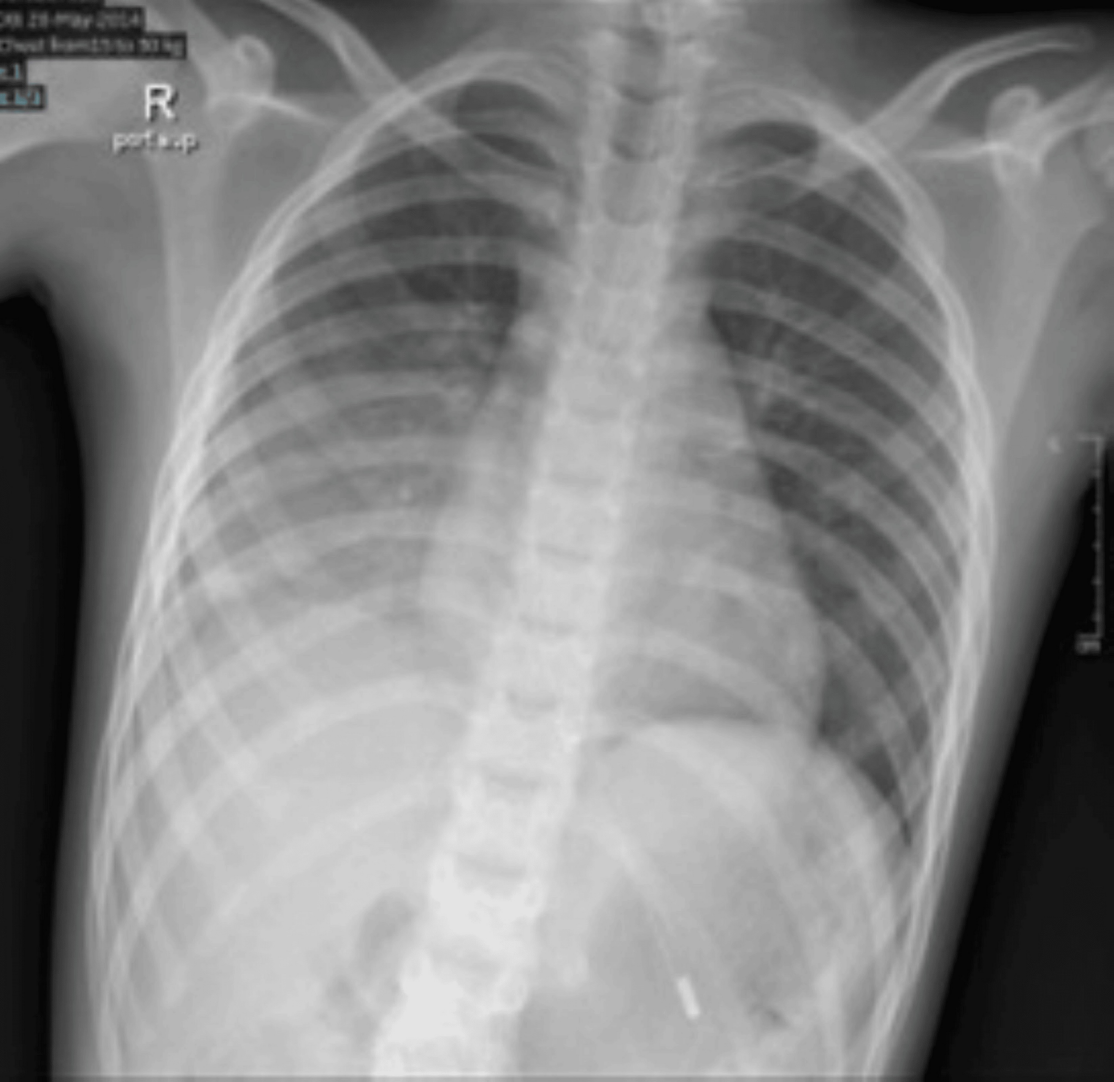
Chest X-ray showing homogeneous opacity in the right lower lung.
